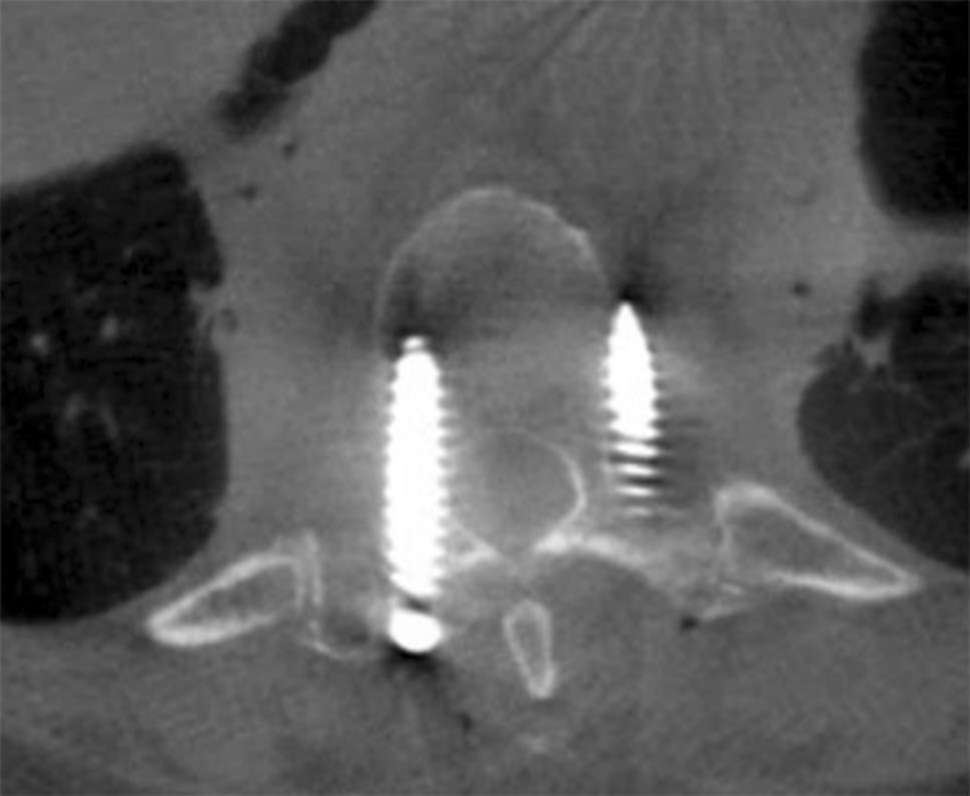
Intraoperative axial CT with lateral breach
